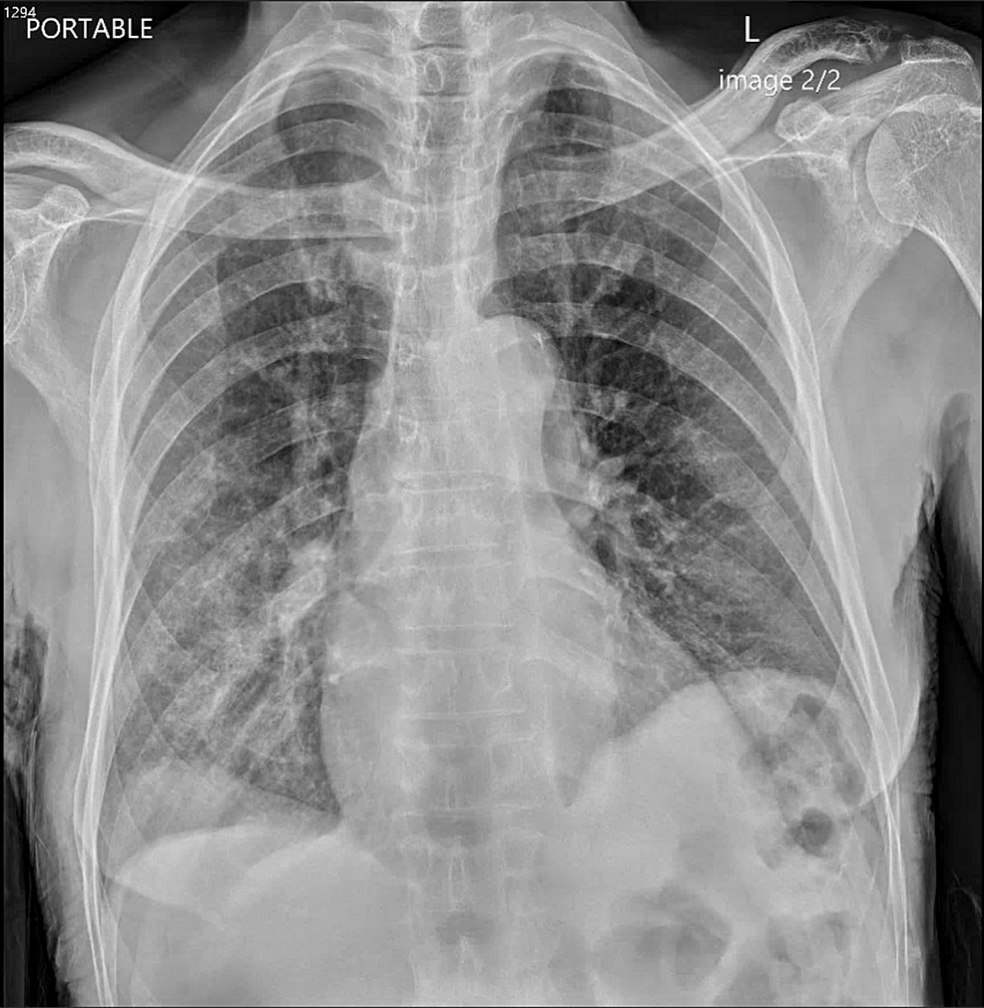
Chest X-ray of our patient on admission
